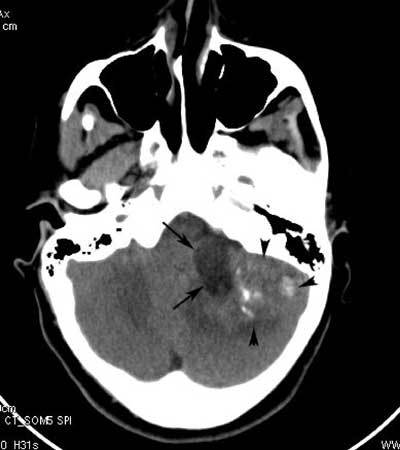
CT scan displays a mass in the left CPA with a cystic (arrows) and a solid component (arrowheads). The solid component of the mass shows dense calcifications.
